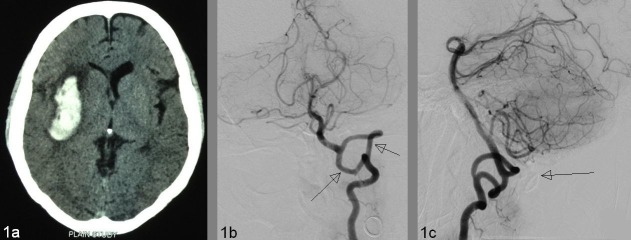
Extracranial vertebral artery fenestration in a 35-year-old hypertensive lady who presented with a right putamen hemorrhage 1A: Acute right putamen hemorrhage in CT scan. 1B: Detection of both widely placed fenestrated segments of VA (arrows) in selective left VA injection (AP view). Length/width of the right limb is 58.7 mm/3.1 mm and the left limb is 62.3 mm/2.9 mm. VA diameters distal to fenestration are 4.0 mm and proximal is 4.3 mm. 1C: Bony landmark of the C1 vertebra (long arrow) in lateral view.
